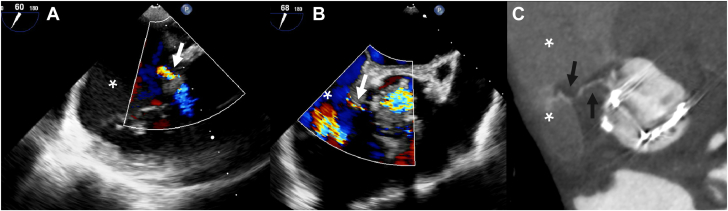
Fistula between the aortic root and right atrium in a patient with aortic biological prosthetic heart valve endocarditis. Fistula between the aortic root and right atrium (asterisks) shown on transesophageal echocardiography with a Doppler jet traversing from the aortic root to the right atrium (A and B) and computed tomography with a contrast-enhanced trajectory between the aortic root and right atrium (arrows) (C).
